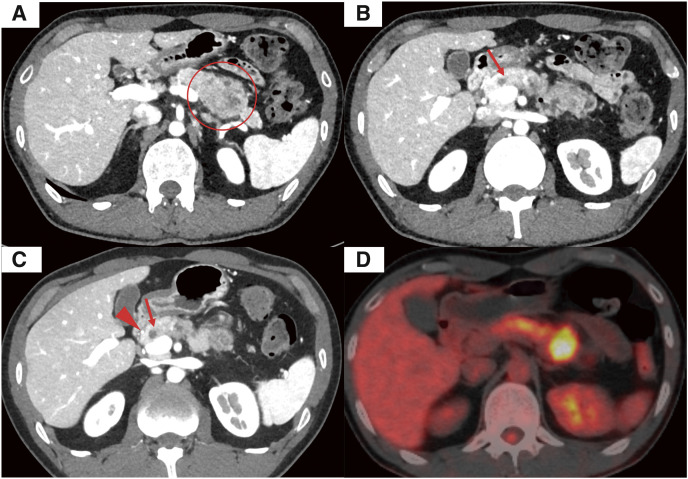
(A) Contrast-enhanced CT showing a well-defined tumor in the pancreatic tail (circle). (B) The tumor extended into the main pancreatic duct, with the progression reaching just above the superior mesenteric vein (arrow). (C) The leading edge of the tumor (arrow) was adjacent to the gastroduodenal artery (arrowhead). (D) FDG positron emission tomography-CT showing increased FDG uptake in the tumor, without lymph node involvement or distant metastases. CT, computed tomography; FDG, fluorodeoxyglucose
